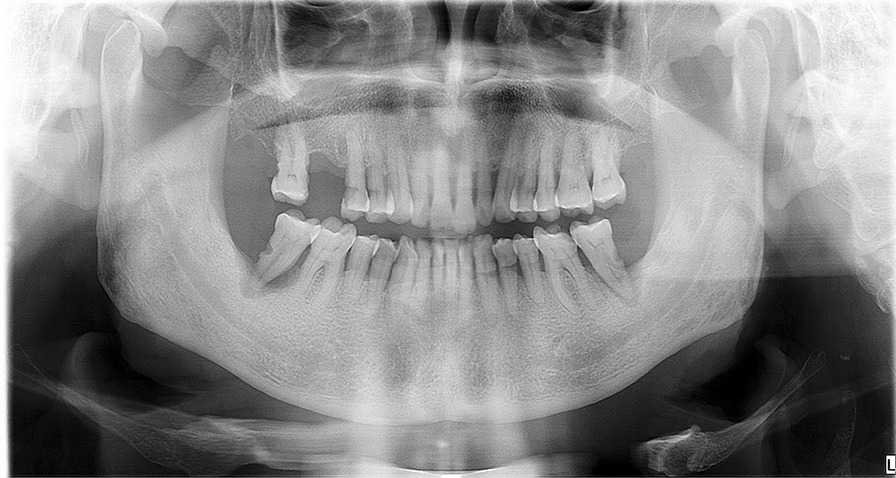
Panoramic radiograph showed no obvious bone resorption except periodontitis
